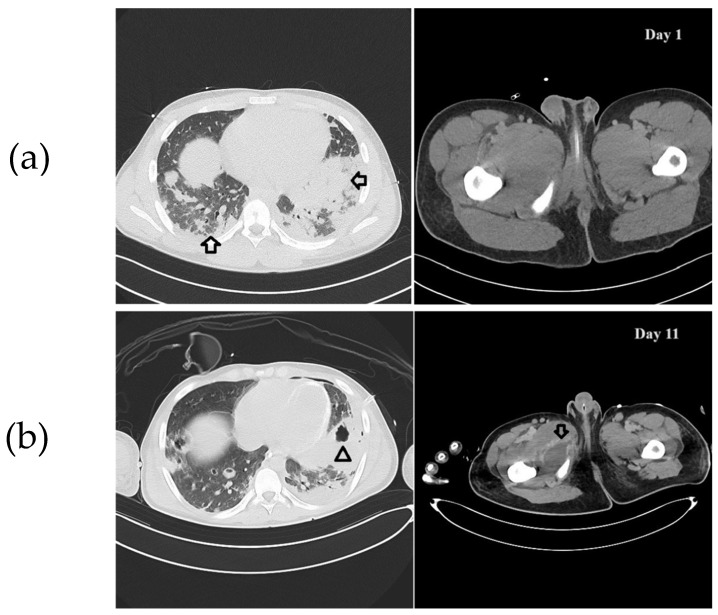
(a) CT scan imaging on day 1. On the left, chest CT. Arrows indicate the abscesses affecting the lung parenchyma. On the right, thigh CT. No abscess is evident. (b) CT scan imaging on day 11. On the left, chest CT. The arrowhead indicates cavitation of an abscess. On the right, thigh CT. The arrow indicates an abscess at the medial region of the thigh.
